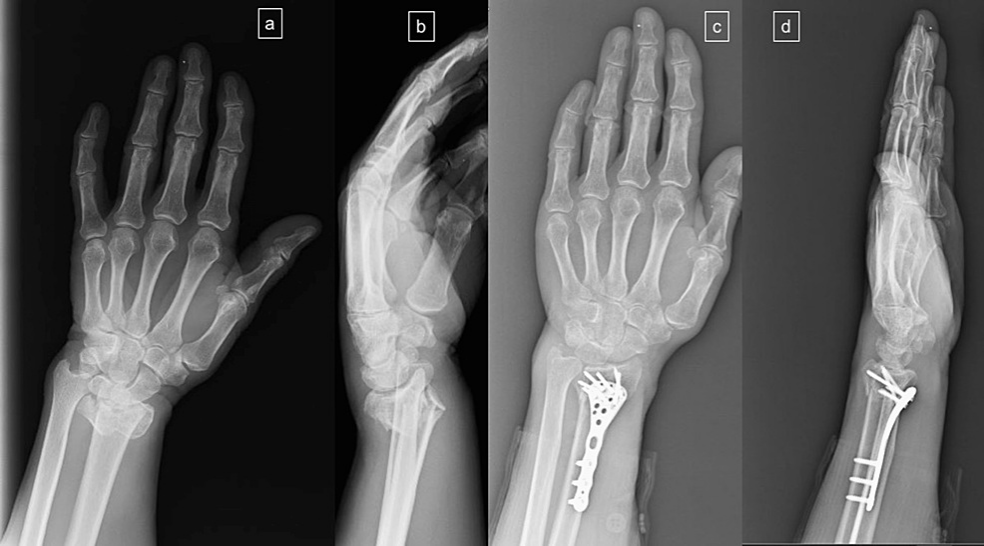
A 68-year-old male with an intraarticular comminuted distal radial fracture treated with a volar locking plate a: Preoperative anteroposterior X-ray of the wrist; b: Preoperative lateral X-ray of the wrist intraarticular and volar metaphyseal fragments; c: Open reduction and fixation with a distal anatomic locking plate d: Lateral X-ray of the wrist
